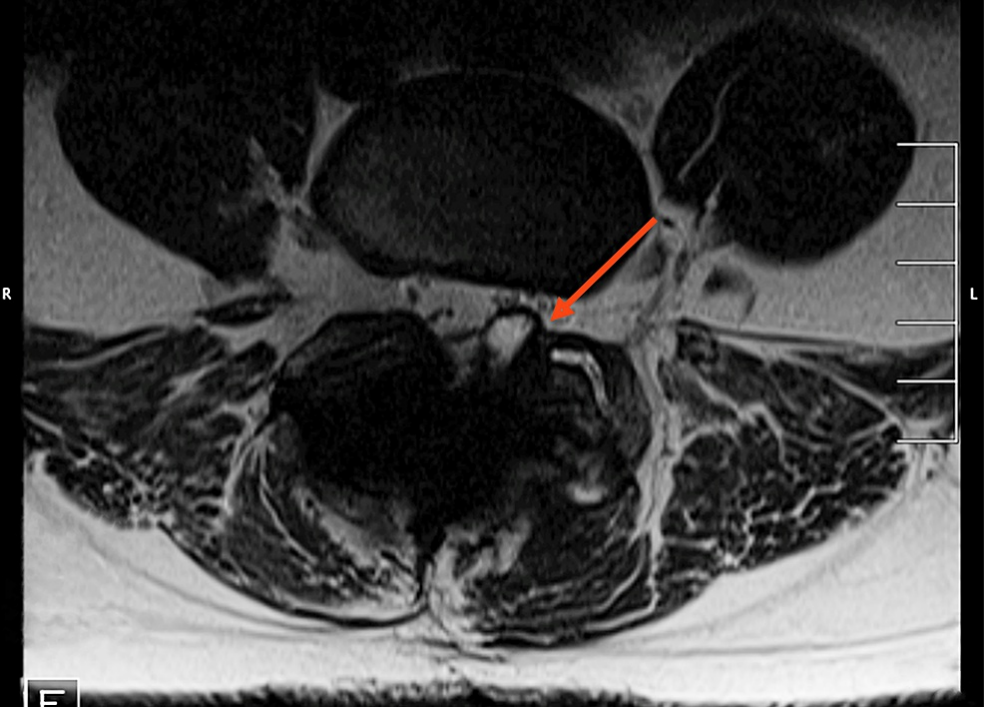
Preoperative axial cross-sectional T2 magnetic resonance imaging of the L4-L5 level. The facet cyst is visible on the left facet joint.
